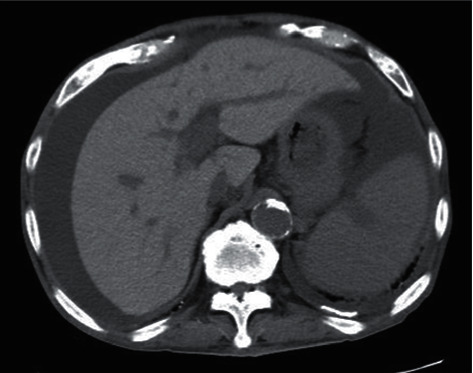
Image of plain computed tomography. Massive ascites and increased liver density.
